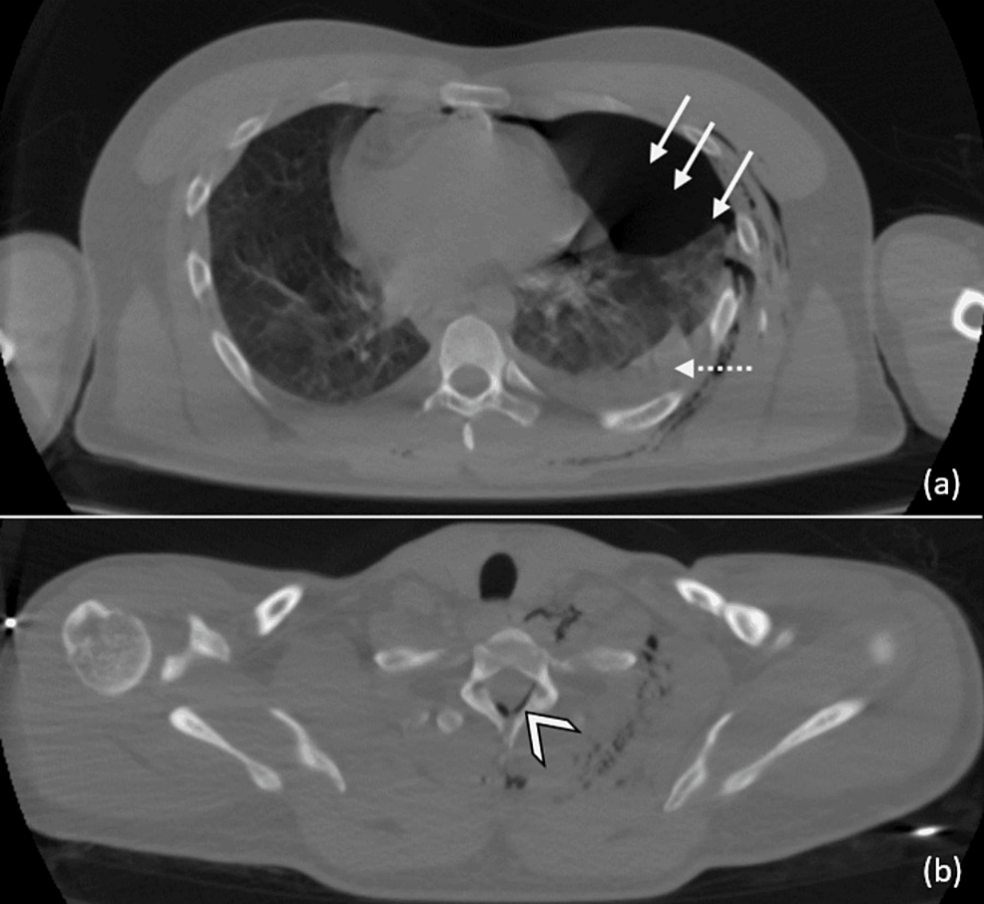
(a) Axial non-contrast chest CT images in the lung window, showing a left pneumothorax (solid white arrows) and minimal left-sided pleural effusion (dashed white arrow). (b) Presence of air within the spinal canal (white arrowhead). CT: computed tomography
